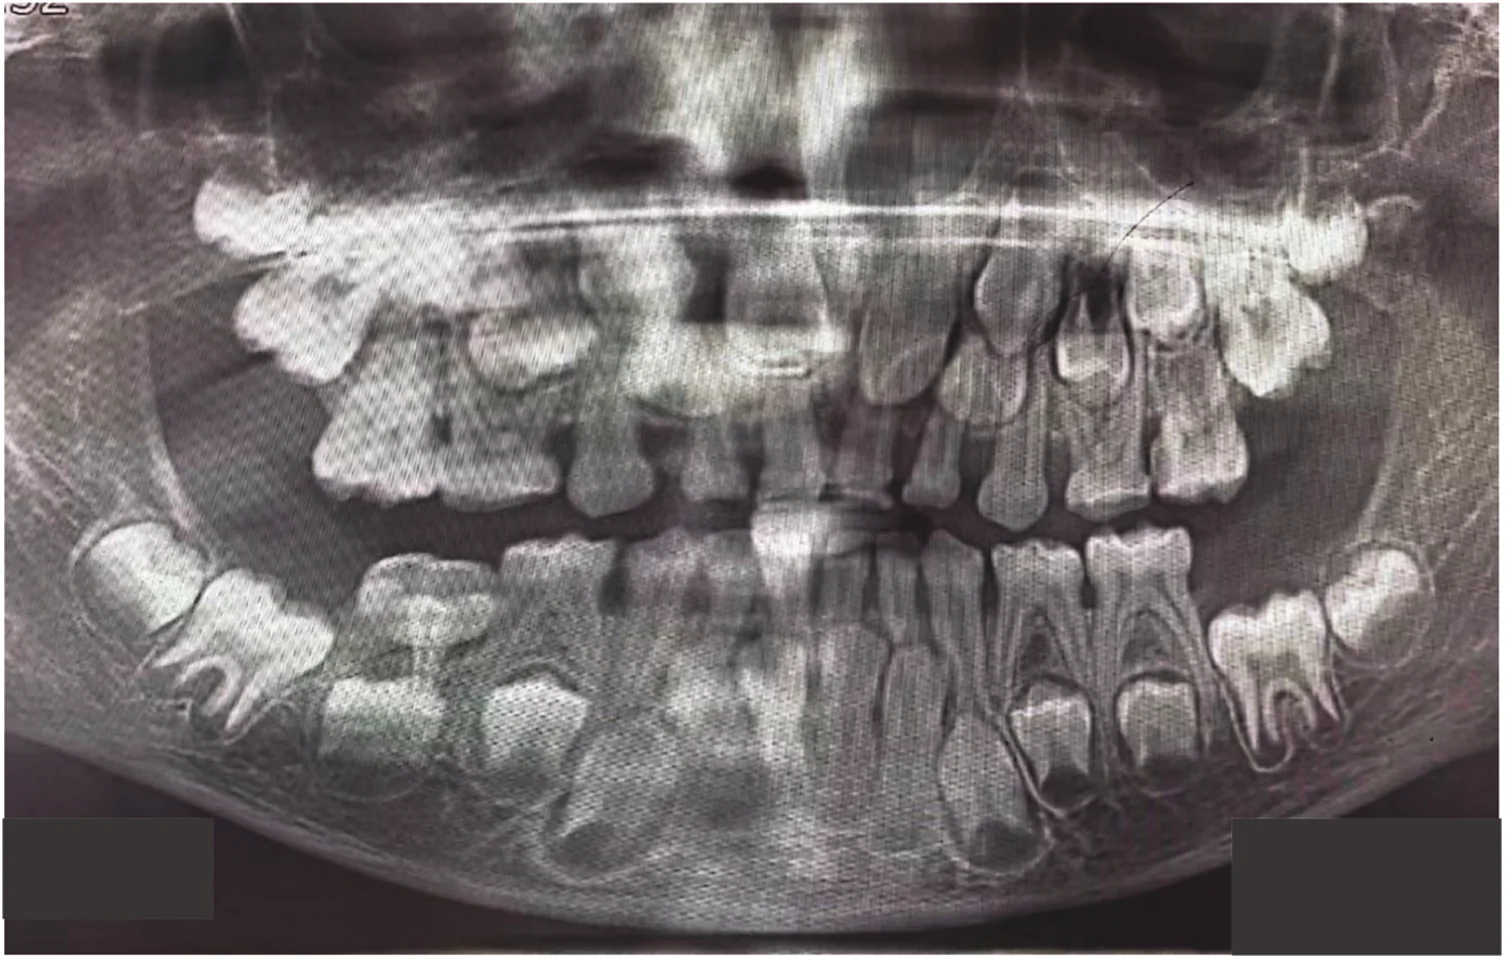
Panoramic radiograph of the dentition. The radiograph shows delayed exfoliation of primary teeth, delayed eruption of permanent teeth, and delayed dental development. Uneven development and abnormal positioning of several maxillary and mandibular teeth are observed. No obvious supernumerary teeth are identified on this radiograph.
